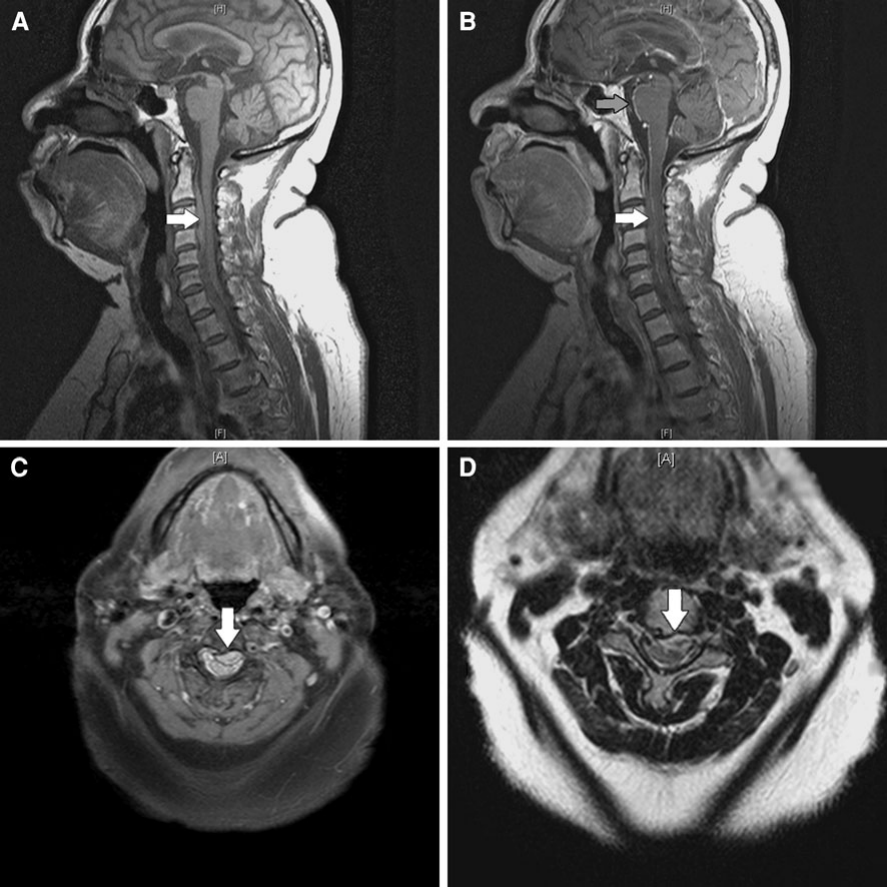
Sagittal pre (a) and post-contrast (b) T1 MRI demonstrate an epidural collection spanning from mid-C2 through T1/T2 vertebral level (*white arrow*). Axial T1 (c) and T2 (d) MRI demonstrate regions of mixed intensity within the cervical epidural collection (*white arrow*), suggestive of spontaneous spinal epidural hematoma (SSEH). A rim of contrast enhancement is seen extending from the upper cervical cord to the ventral medulla and anterior pons (b, *gray arrow*)
